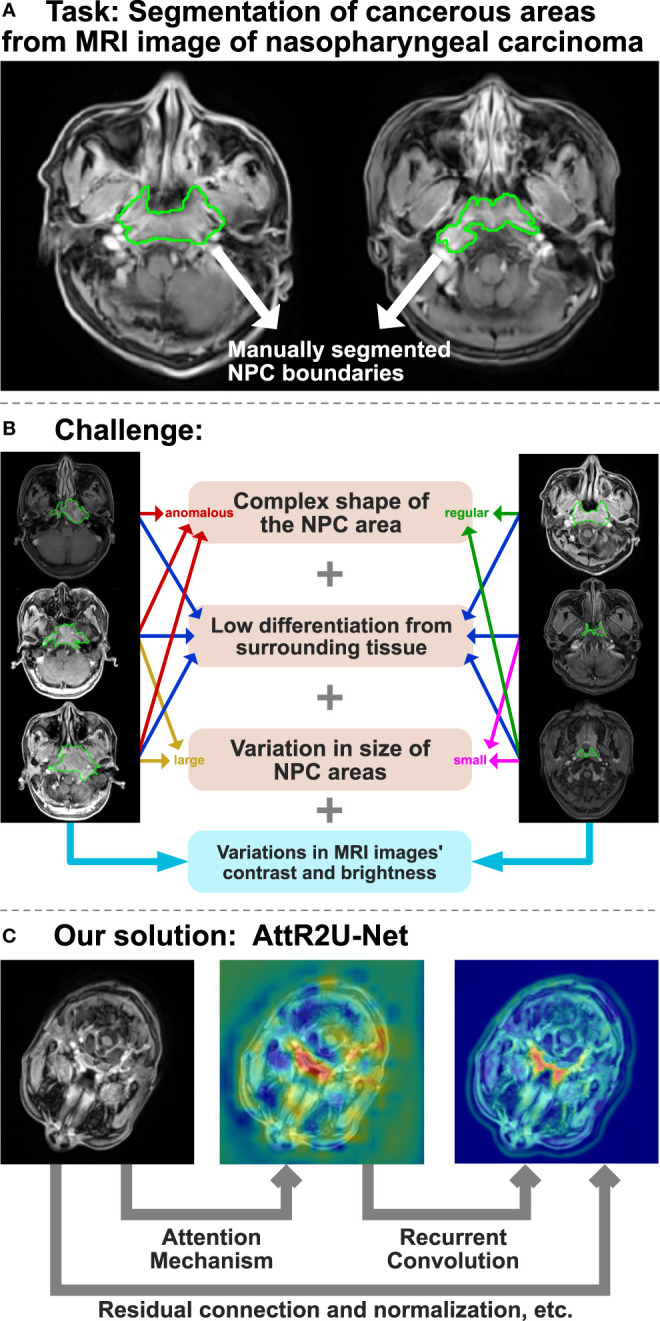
The overview of AttR2U-Net model for automatic segmentation of nasopharyngeal carcinoma. (A) The task of the AttR2U-Net model. (B) The challenges of segmentation arise from two main aspects, in which orange represents NPC internal causes and blue represents external cause. (C) The four main mechanisms of our solution: attention mechanism to localize NPC accurately, recurrent convolution to extract boundary details precisely, residual connection to build a deeper and more efficient model, and normalization to reduce the variations in MRI images.
